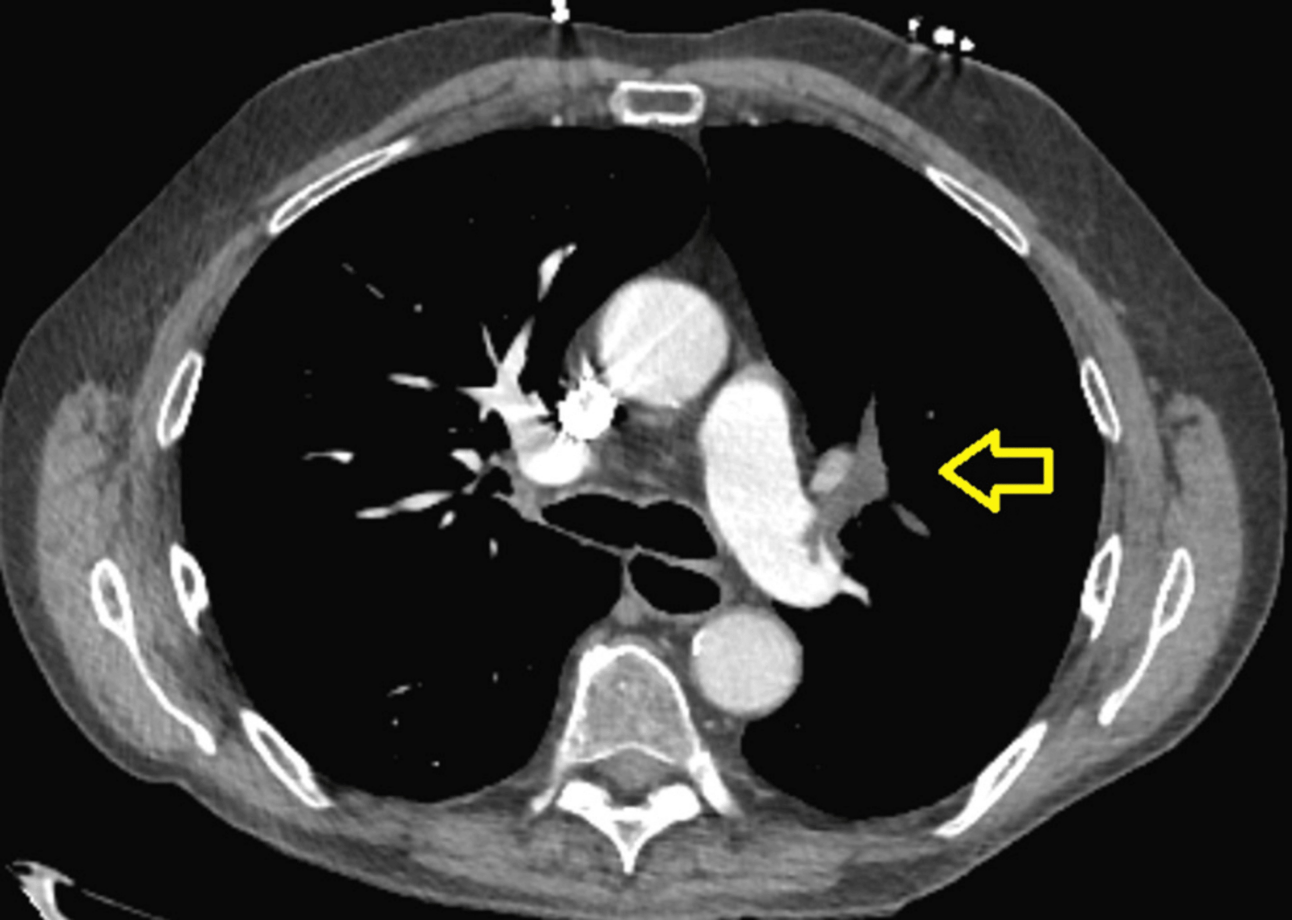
A CT angiogram of the chest The scan revealing a moderate-sized acute pulmonary embolus involving the left upper lobe branches of the pulmonary arterial system measuring 3 cm in length and 0.8 cm in diameter. There were smaller and more peripheral emboli within the sub-segmental branches in the upper lobes bilaterally.
